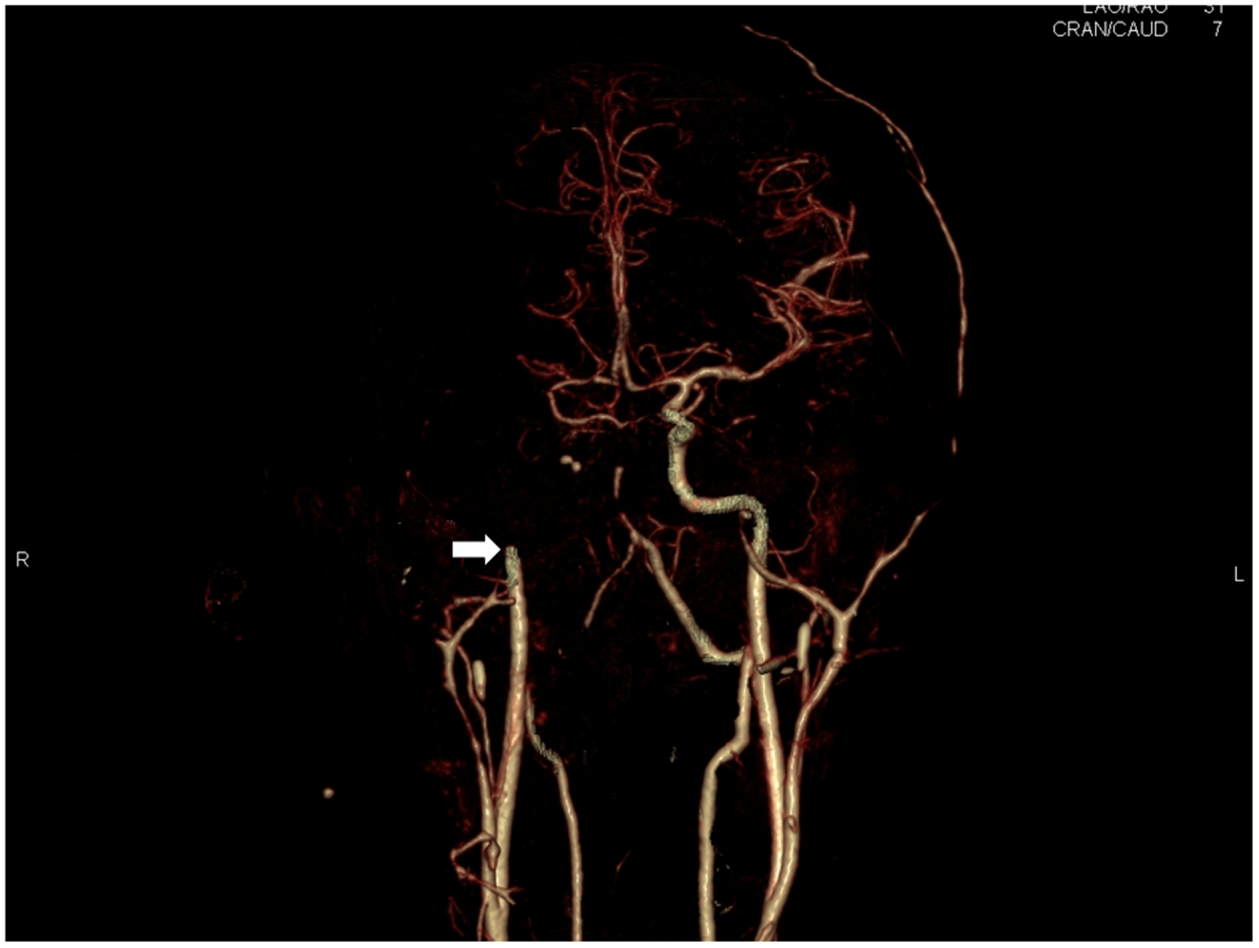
3D reconstruction of - cranial CT-Angiography after bone-removal. The white arrow shows the occlusion of the right internal carotid artery (white arrow) and the lack of vessel opacification in the right hemisphere.
